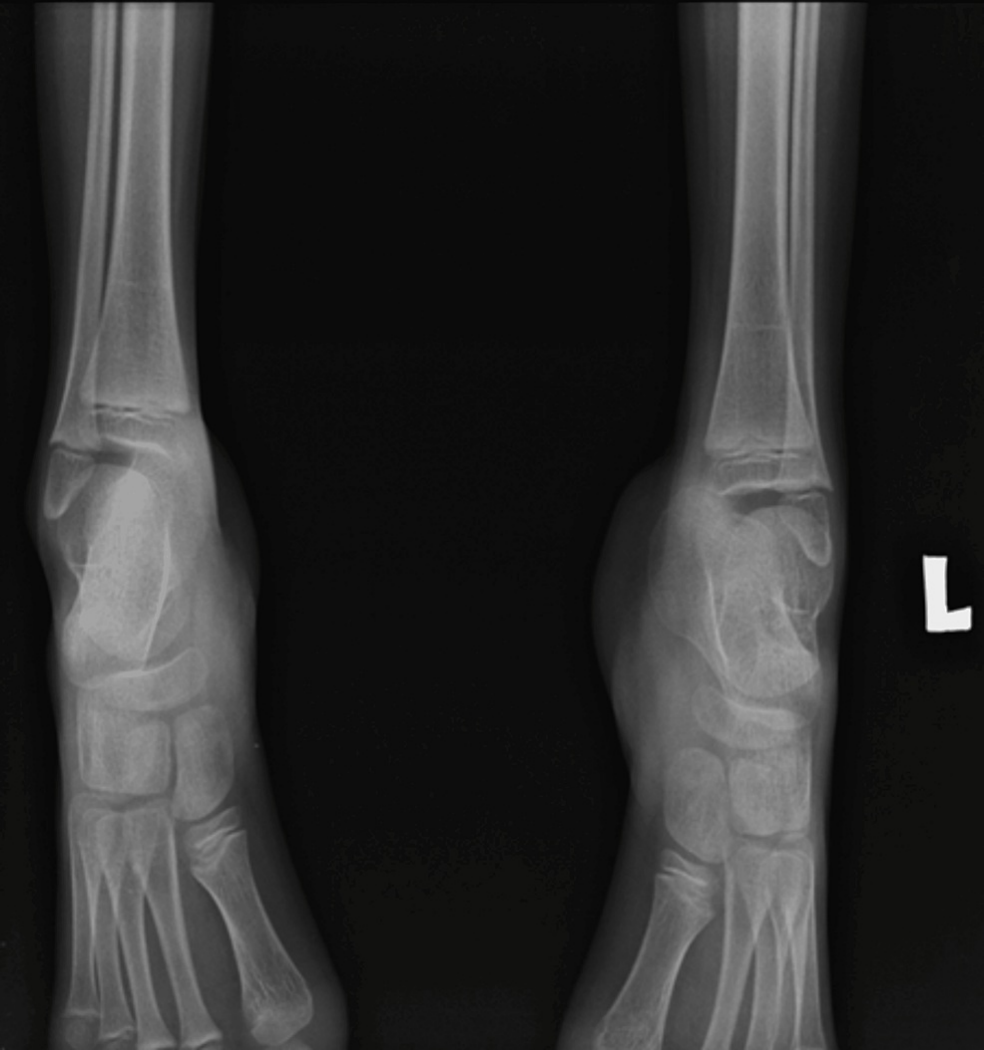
Radiographical images of the bilateral foot showing no bony deformity of the bilateral foot
